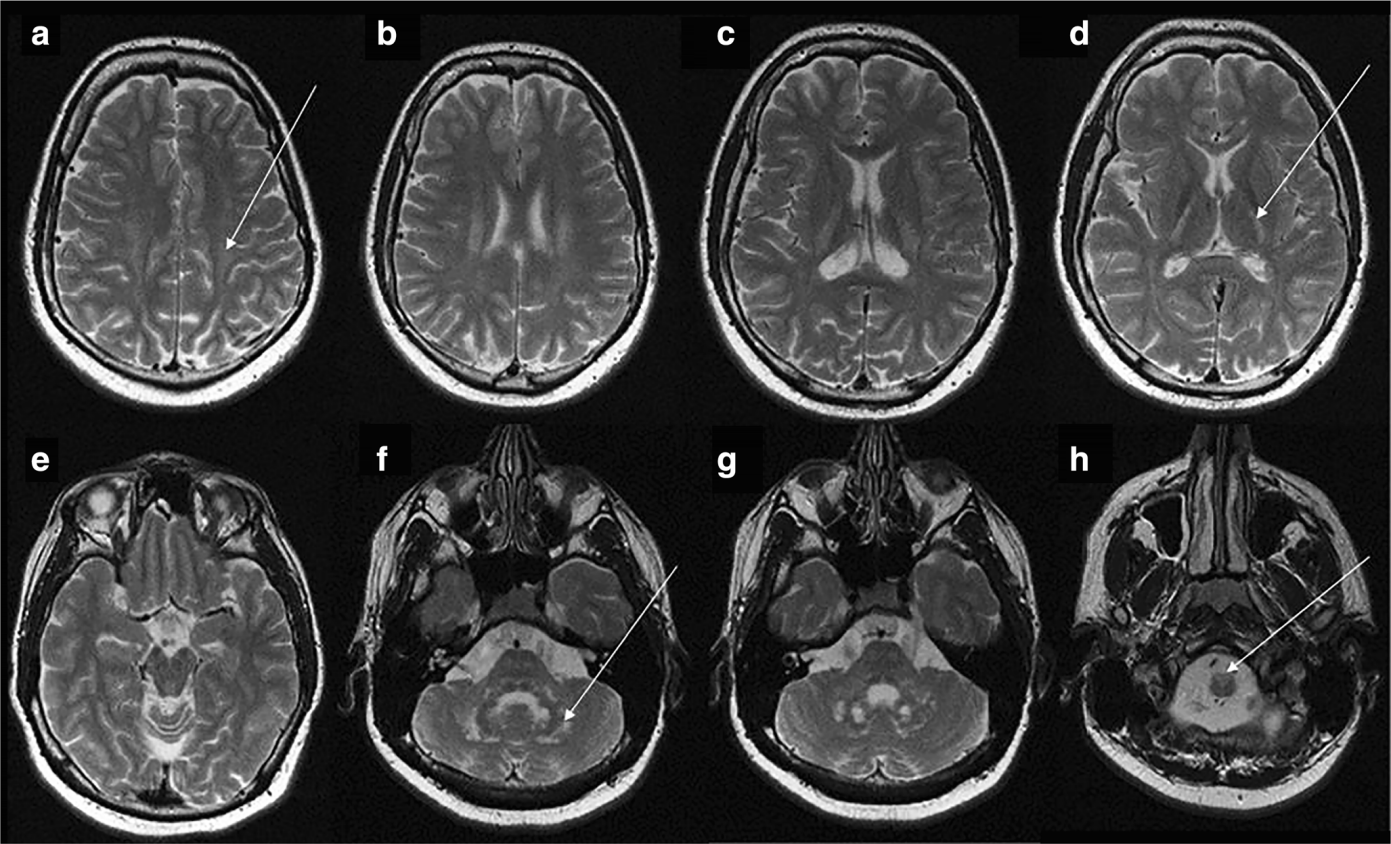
MRI examination at the age of 21 years. Axial T2-weighted images. See the bilateral hyperintense signal of the posterior part of the centrum semiovale (arrow on a), posterior limb of internal capsule (arrow on d), cerebellar white matter (arrow on f). The antero-medial part of medulla oblongata is also hyperintesive (arrow on h)
